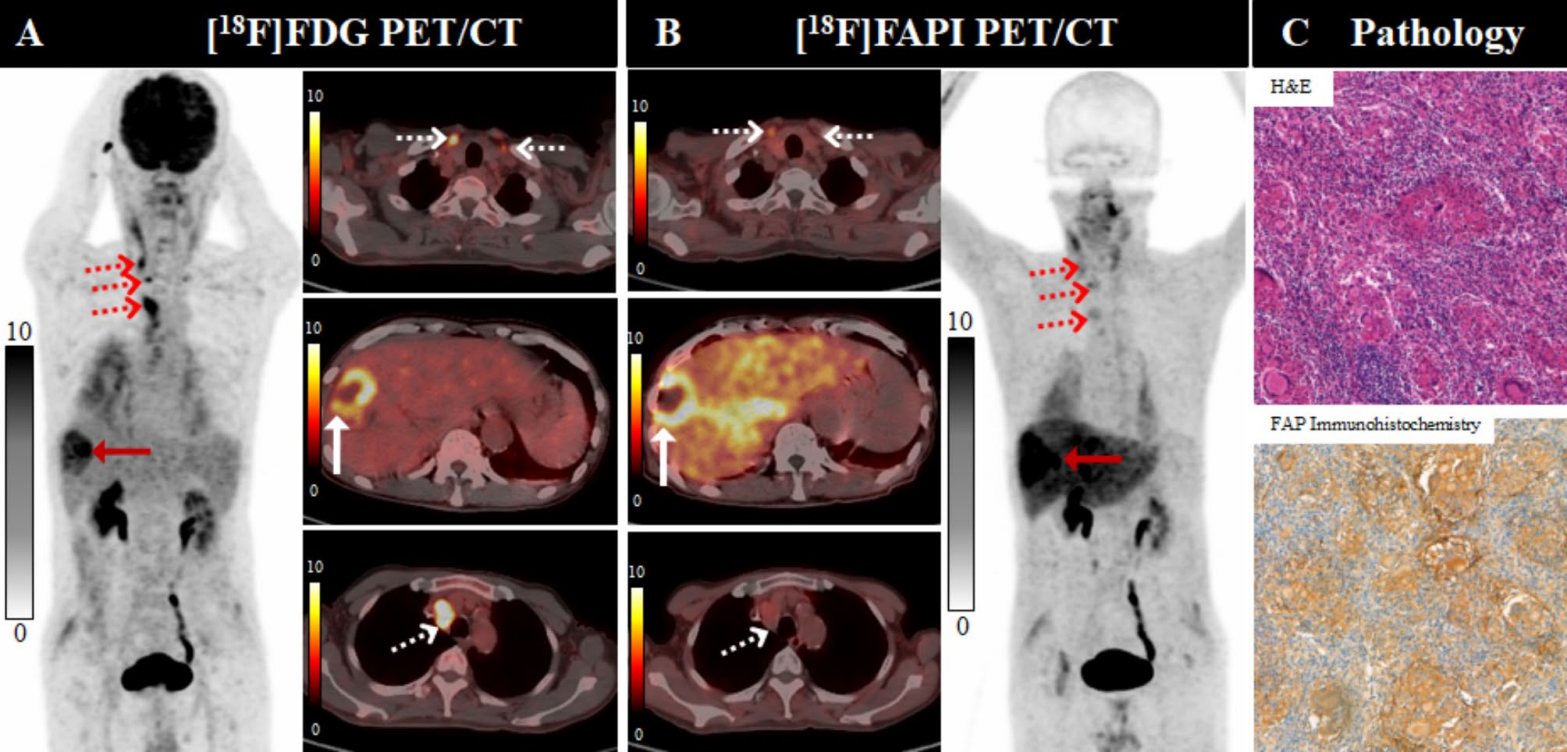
A 70-year-old male patient (Patient No. 19) with intrahepatic cholangiocarcinoma (ICC) was confirmed by biopsy. Compared with [^18^F]FDG PET/CT (A), [^18^F]FAPI-04 PET/CT (B) showed significantly increased radiotracer uptake in the primary tumor (white arrow in axial images), yet displayed elevated hepatic [^18^F]FAPI-04 activity in the liver parenchyma background. There were several lymph nodes (dotted arrows), follow-up evaluations confirmed reactive. Pathological findings (C) indicate a heightened expression for FAP
